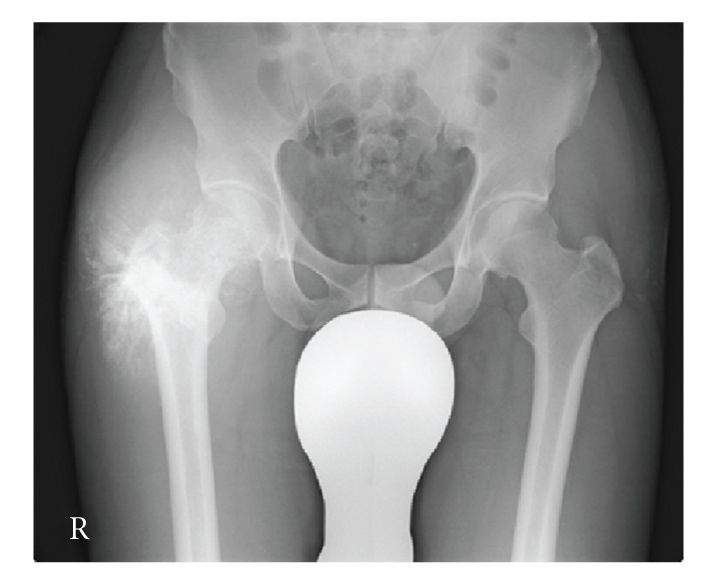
Plain radiography at first visit. Marked calcification was observed in the right thigh.
